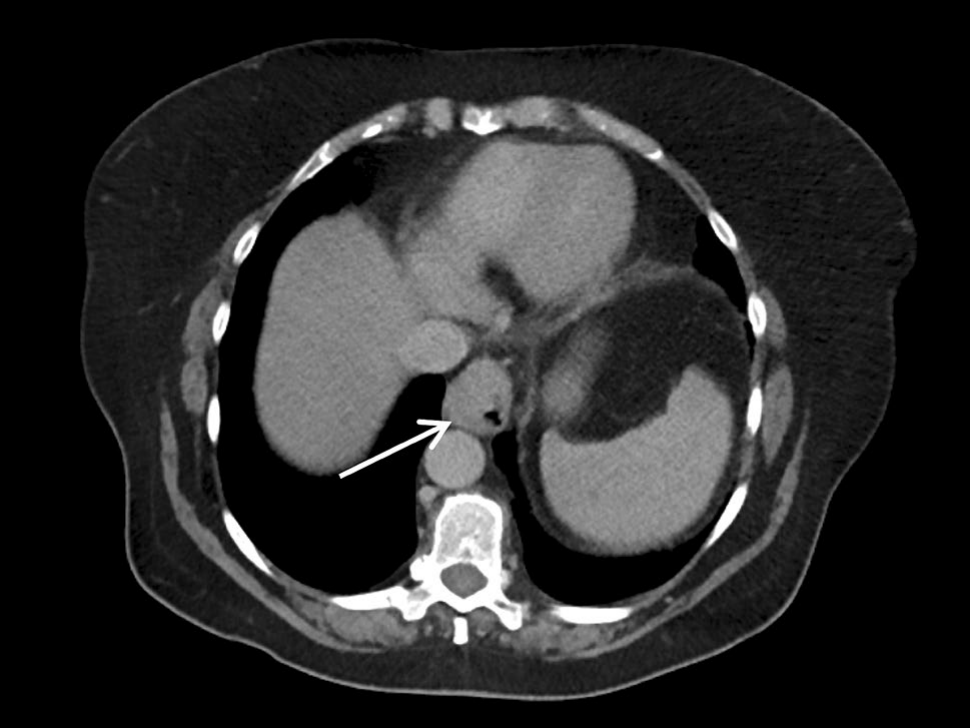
CT scan showing oesophageal cancer
